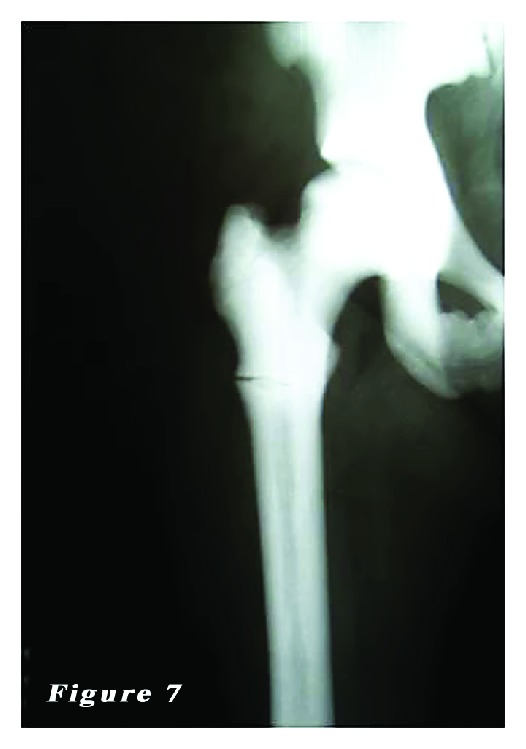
24-year-old female patient (second case) preoperative radiograph of the right femur subtrochanteric stress fractures.
